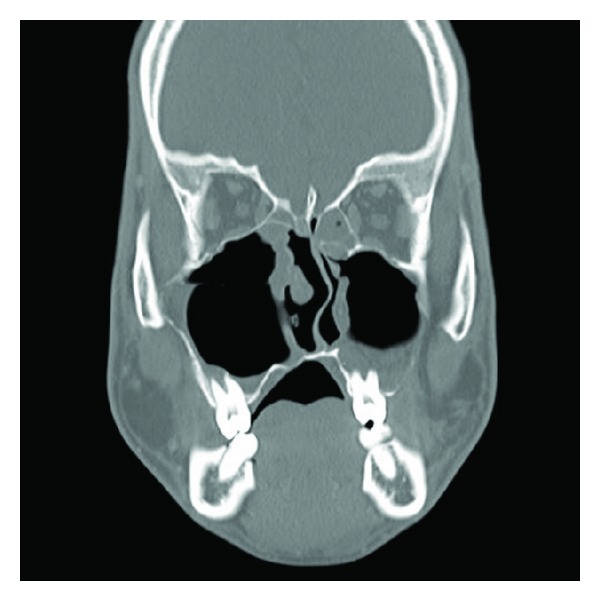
A postoperative coronal computed tomography scan shows that the right maxillary cyst has been removed and obstruction of the inferior nasal meatus has resolved completely.
